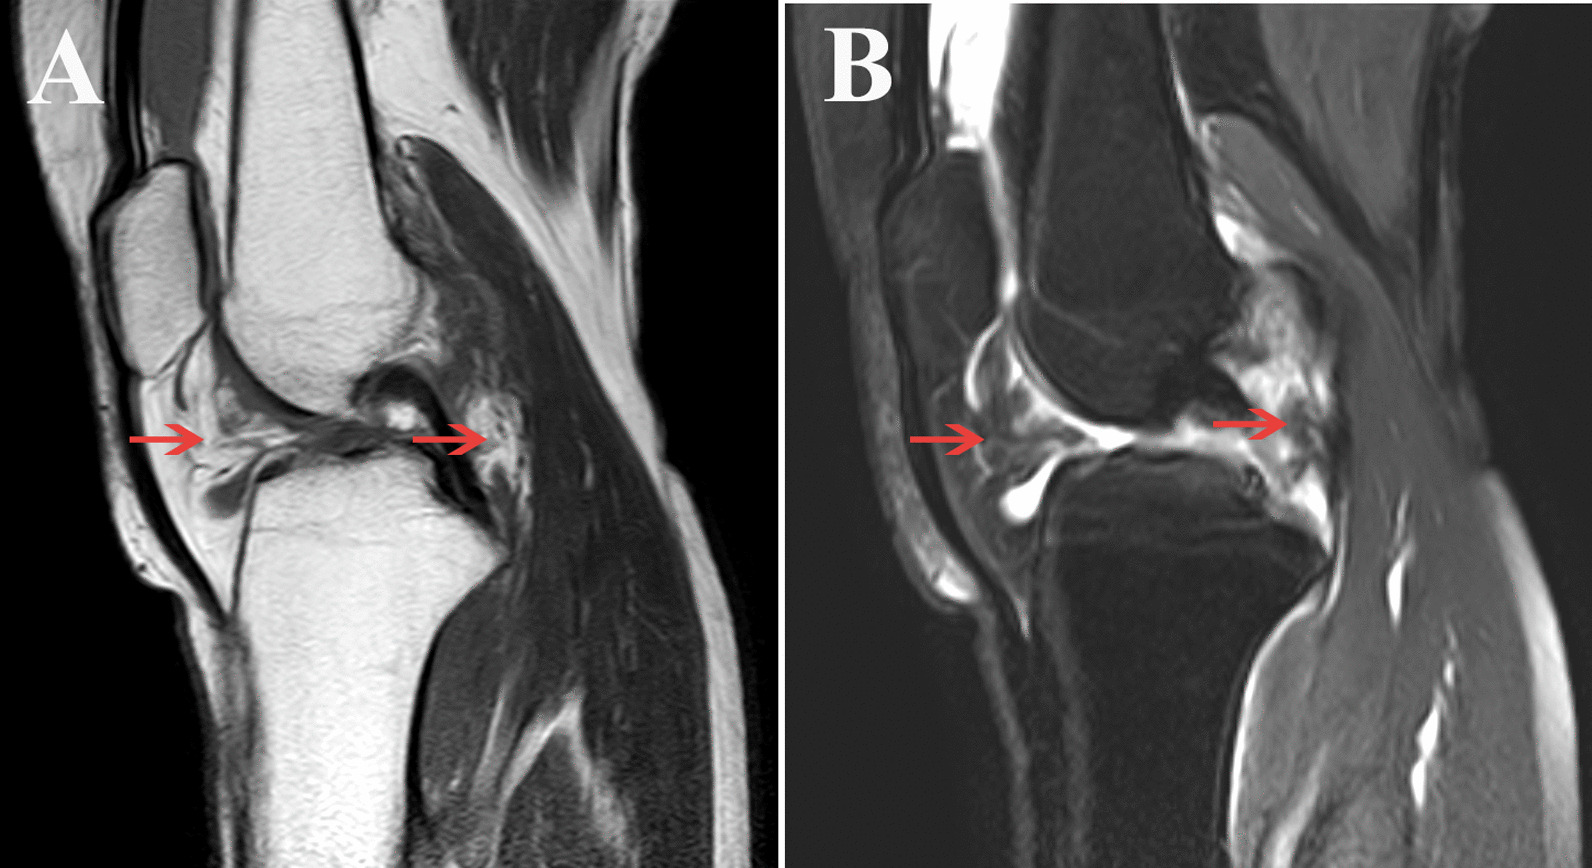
Preoperative MRI showed that the synovial membrane of the knee joint was significantly thickened, showing nodular low T2WI and T1WI low signal changes. A. T1WI, B. T2WI. Red arrows indicate mainly located in the infrapatellar fat pad and next to the cruciate ligament
